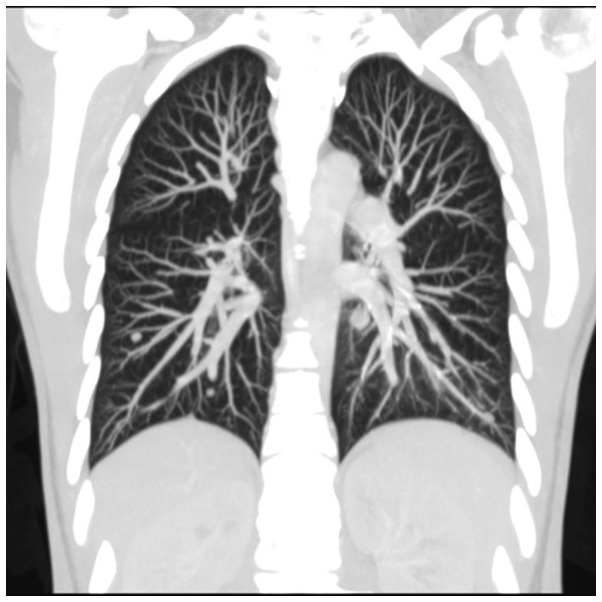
Computed tomography scan of the chest in coronal section showing metastatic lesions.
